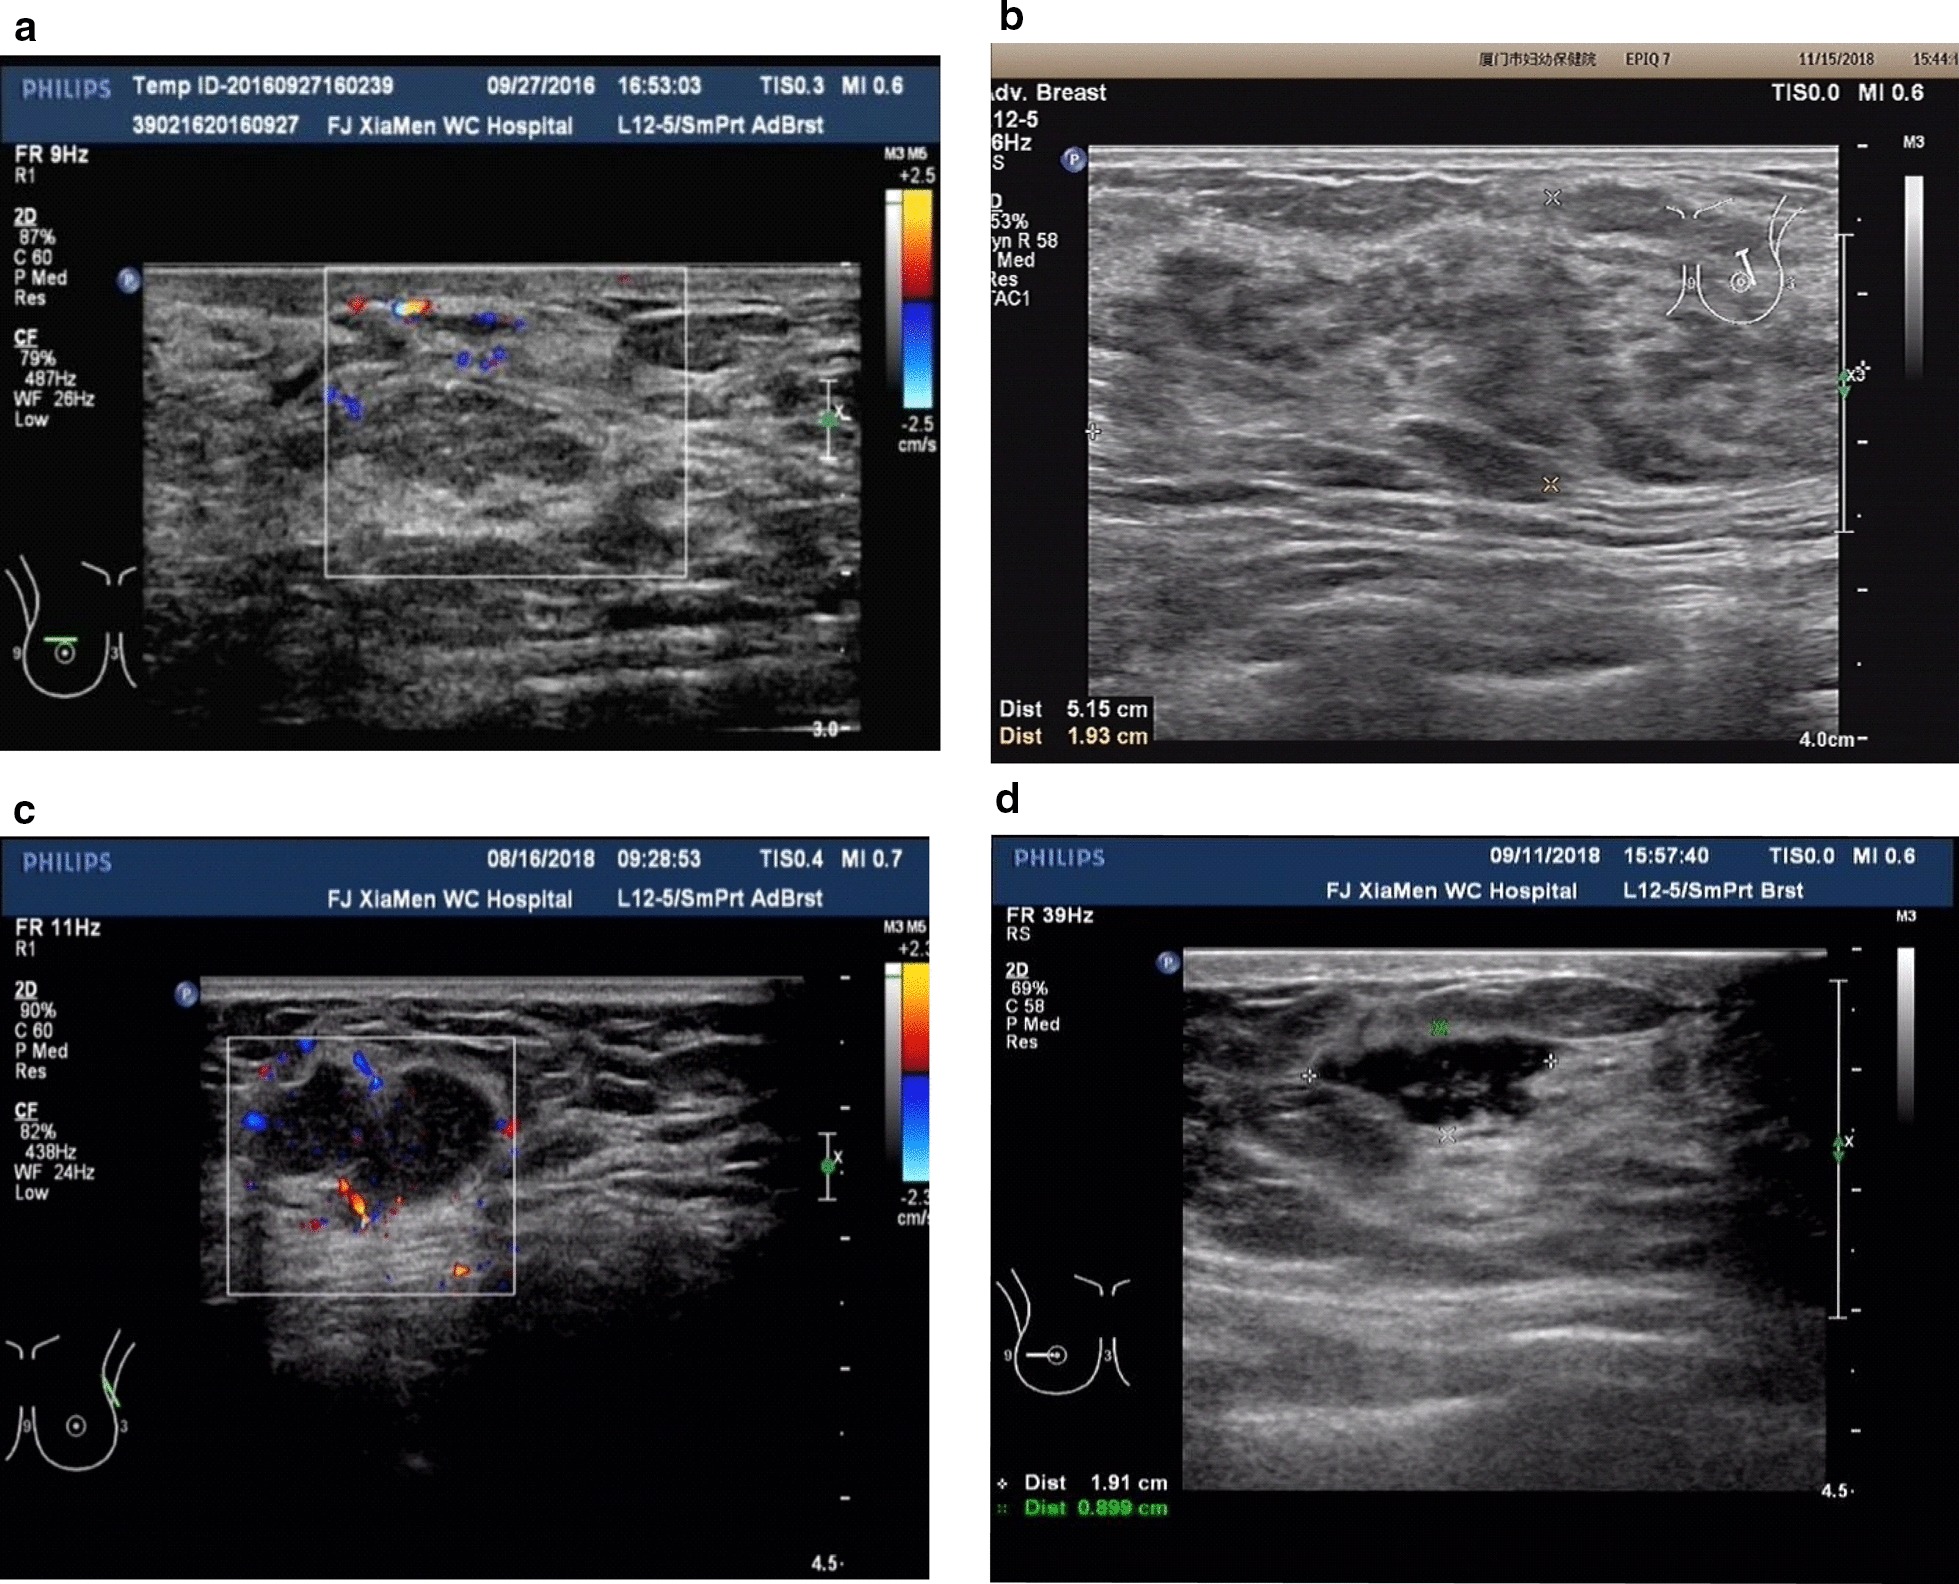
Analysis of the results of ultrasound classification of lesions. a A mixed type of diffuse abscess. Large patchy hypoechoic or mixed echo areas scattered; glands and multiple abscess cavities under the skin form tunnels; b a sheet hypoechoic type. Sheet hypoechoic area showed unclear borders, disordered structure, and no abscess cavity; c a localized abscess type. There were multiple abscesses with clear borders; d a localized hypoechoic mass type. The echo was uneven and irregular, but with clear borders
